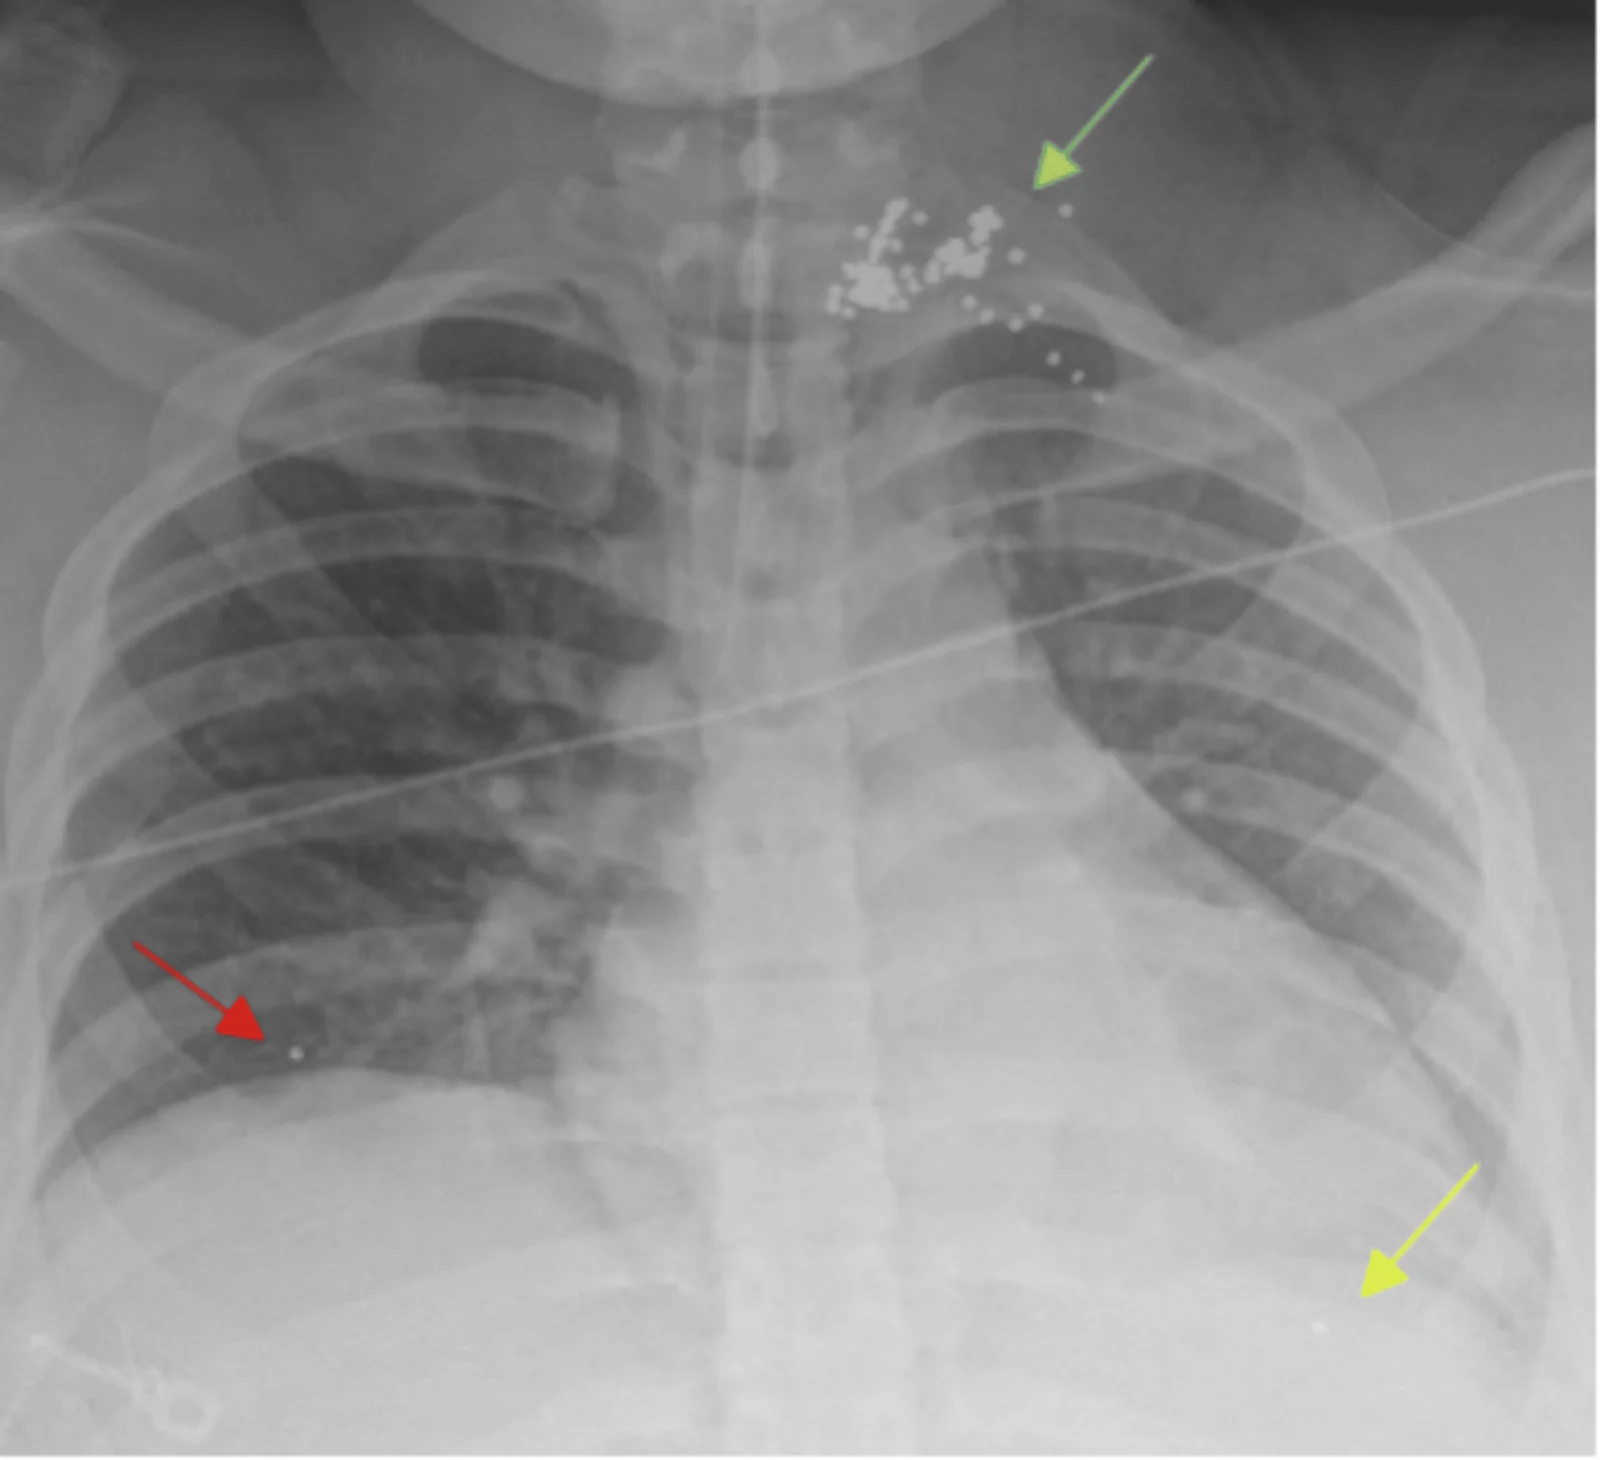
Chest X-ray in the trauma bay showing birdshot in the neck (green arrow), apex of the heart (yellow arrow), and right lung periphery (red arrow).
